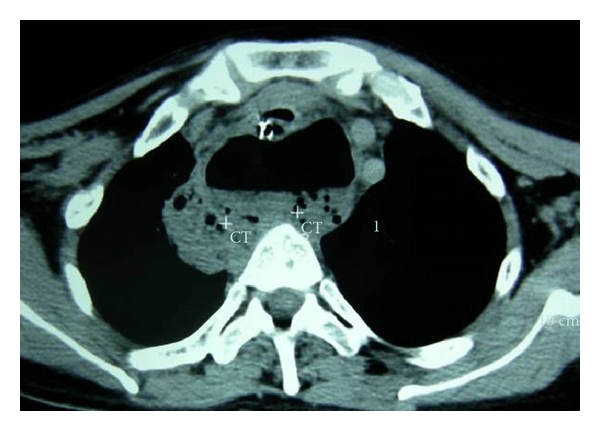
Large superior mediastinal abscess with multiple air pockets on CT scan.
